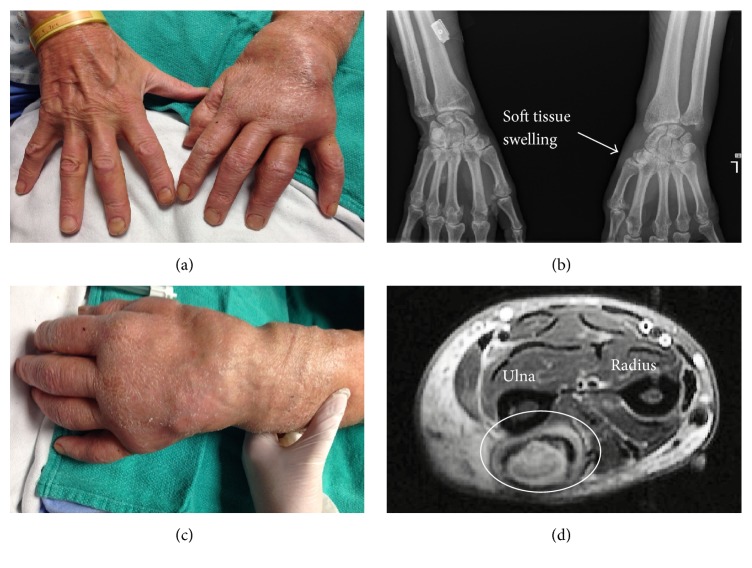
Sporotrichoid spreading of* M. marinum* via lymphatic and myotendinous spreading in disseminated disease. (a) Unilateral granulomatous disease with soft tissue swelling due to lymphatic obstruction, (b) radiograph demonstrating unilateral left soft tissue swelling in absence of erosive disease, (c) dorsal aspect of distal left forearm with visible nodules, and (d) MRI demonstrating myotendinous necrotic granuloma (white circle) in distal aspect of extensor carpi ulnaris.
